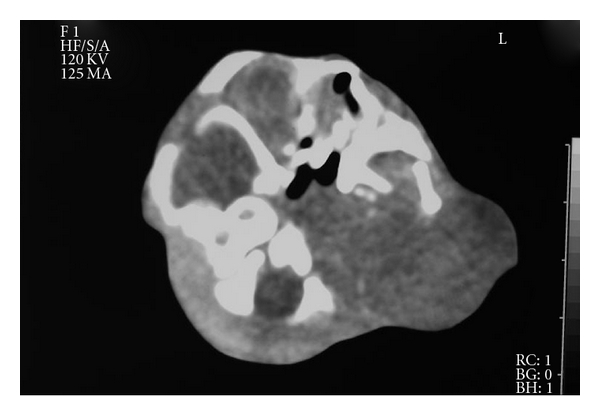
CT image of the cervical mass of aborted fetus.
